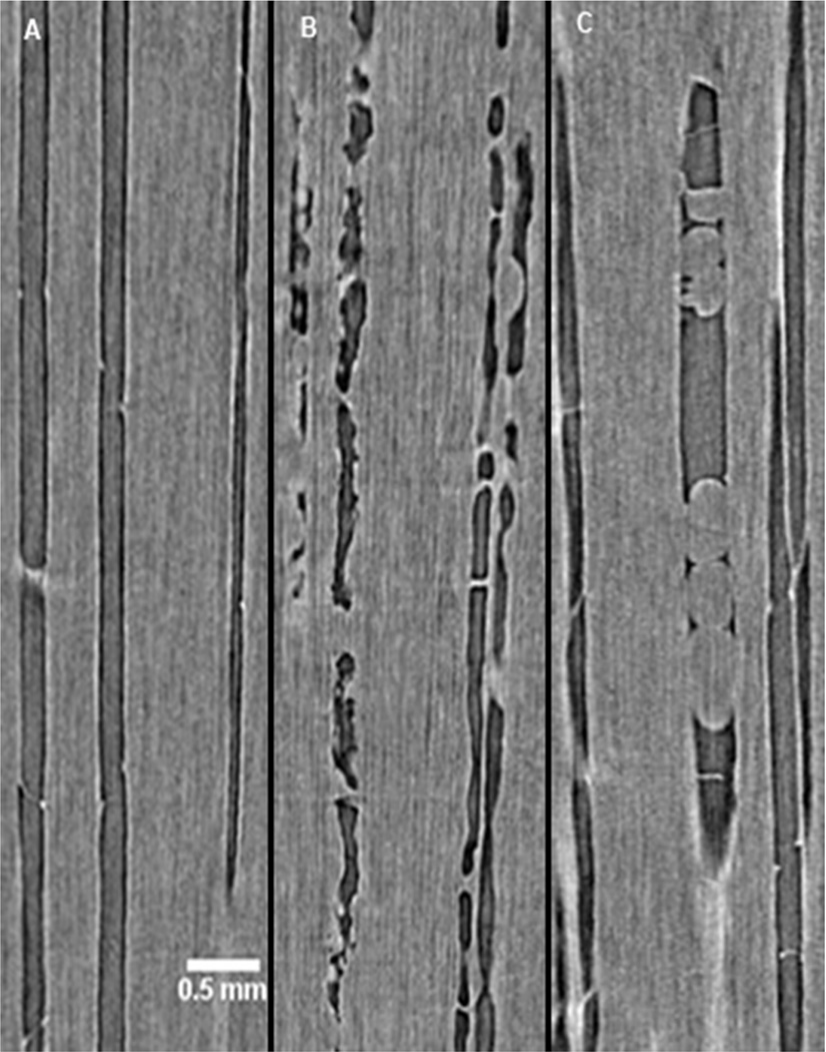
HRCT distinguished gel and tyloses-occluded xylem vessels. Shown here are optical sections of plants representative of three treatment-time combinations. (A) NINW-0 MPI, with unoccluded vessels. (B) IW-2 MPI, with gel-occluded vessels. (C) NIW-2 MPI, with tyloses-occluded vessels. Three-dimensional projections from which these optical sections originated are shown in S1 Video.
